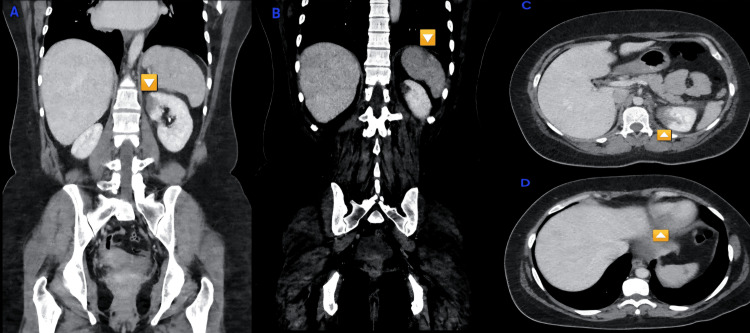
Multimodality imaging findings (A, B) Contrast-enhanced CT demonstrating a left renal infarct. (C) Contrast-enhanced CT showing a splenic infarct. (D) CT angiography highlighting a splenic perfusion defect consistent with infarction. CT: computed tomography
